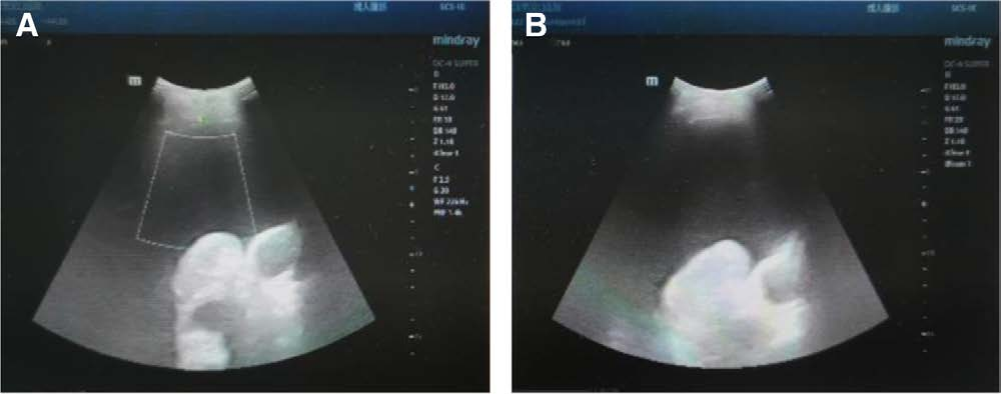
Ultrasound examination of pleural effusion. (A and B) Ultrasound examination indicated a large amount of pleural effusion on the left side.
